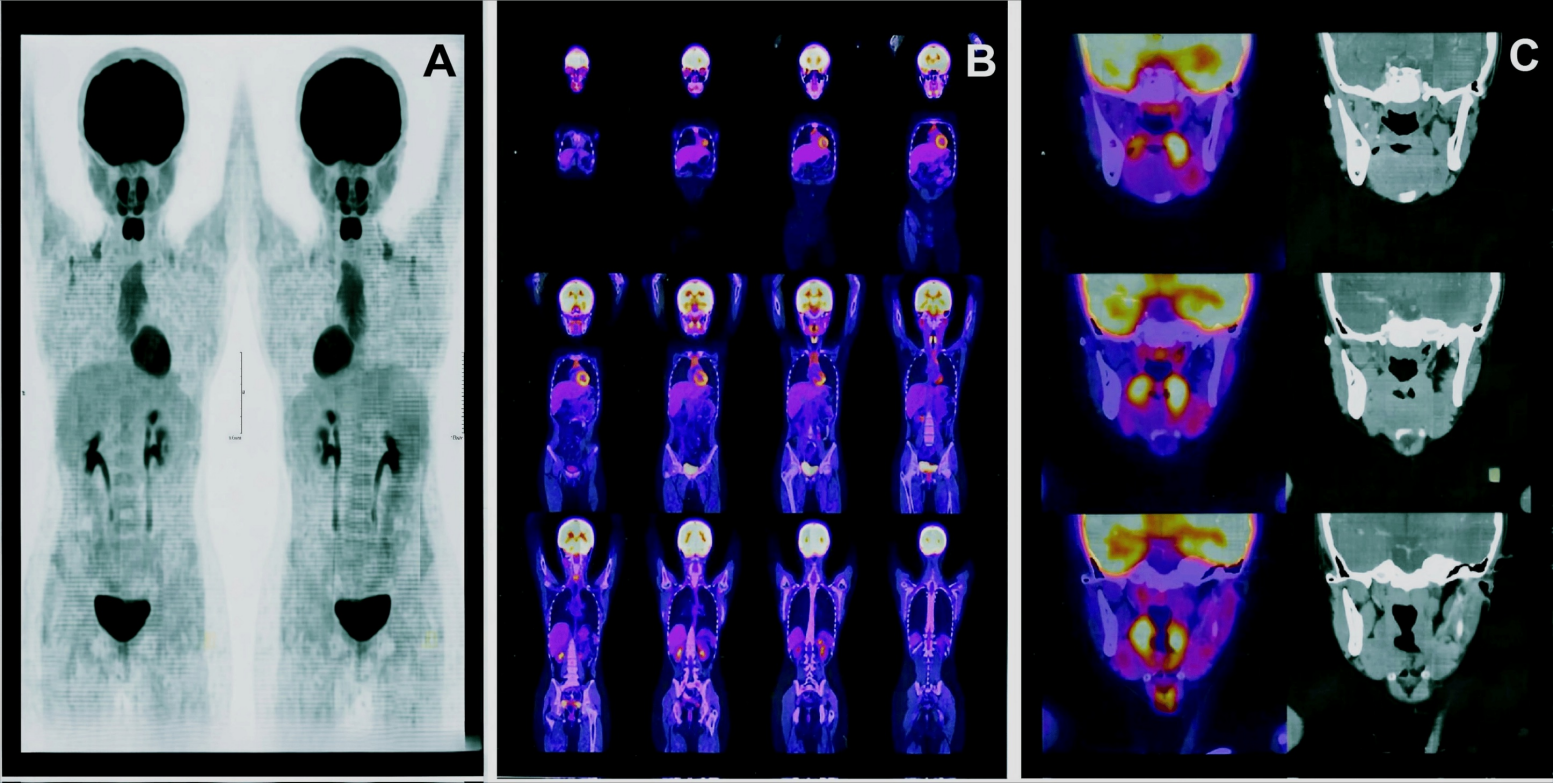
Follow‑up whole‑body and regional PET/CT imaging demonstrating absence of metabolically active disease. Panel A shows paired whole‑body PET maximum‑intensity projections. Panel B presents sequential coronal PET/CT fusion slices from head to pelvis. Panel C displays fused PET/CT and corresponding CT images of the head and neck. The follow‑up PET‑CT scan revealed no pathological metabolic uptake at the surgical bed, within the cervical lymph node chains, or at distant sites, indicating no evidence of metabolically active tumor disease. These findings support a favorable prognosis, for which periodic clinical and imaging surveillance is advised.
